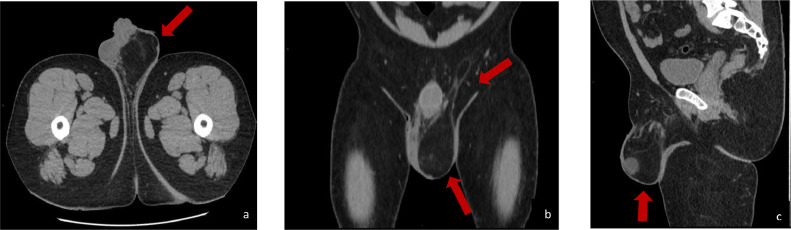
Transversal (A) coronal (B) and sagittal (C) CT scan of the mass of the left spermatic cord, which continued cranially with an adipose “cylinder” along the anatomical vector of the spermatic cord for a length of about 9 cm, of diameters 5.4 cm (lateral-lateral) × 8 cm (antero-posterior).
